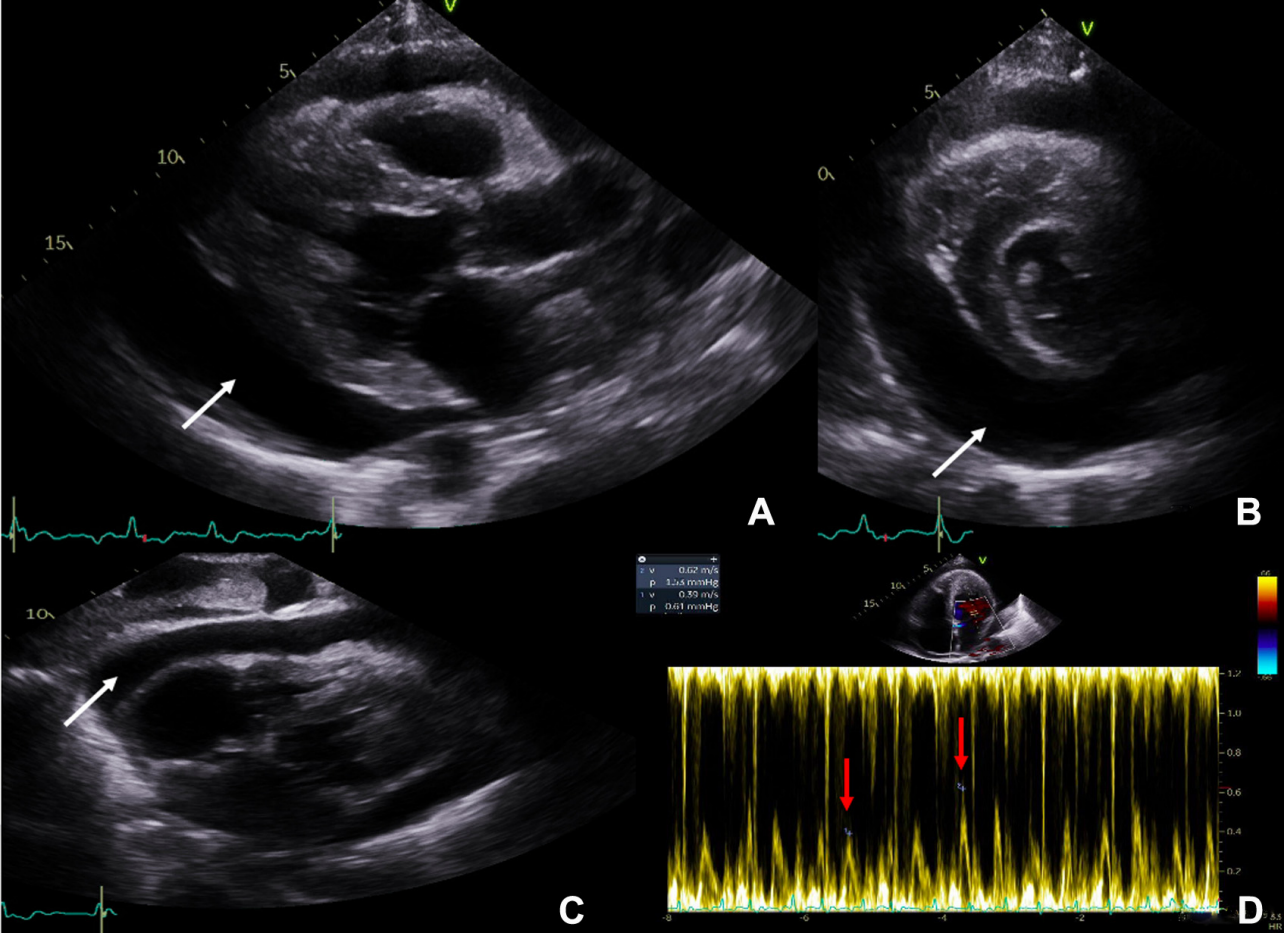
Subsequent Urgent Transthoracic Echocardiogram Urgent limited transthoracic echocardiogram with a large pericardial effusion (white arrows) with right ventricular diastolic collapse and respiratory flow variation across the mitral valves (red arrows, variation of 37%; a respirometer was not used in the emergency room settings). (A) Parasternal long-axis view. (B) Parasternal short-axis view. (C) Subcostal views. (D) Transmitral flow variation during respiration.
